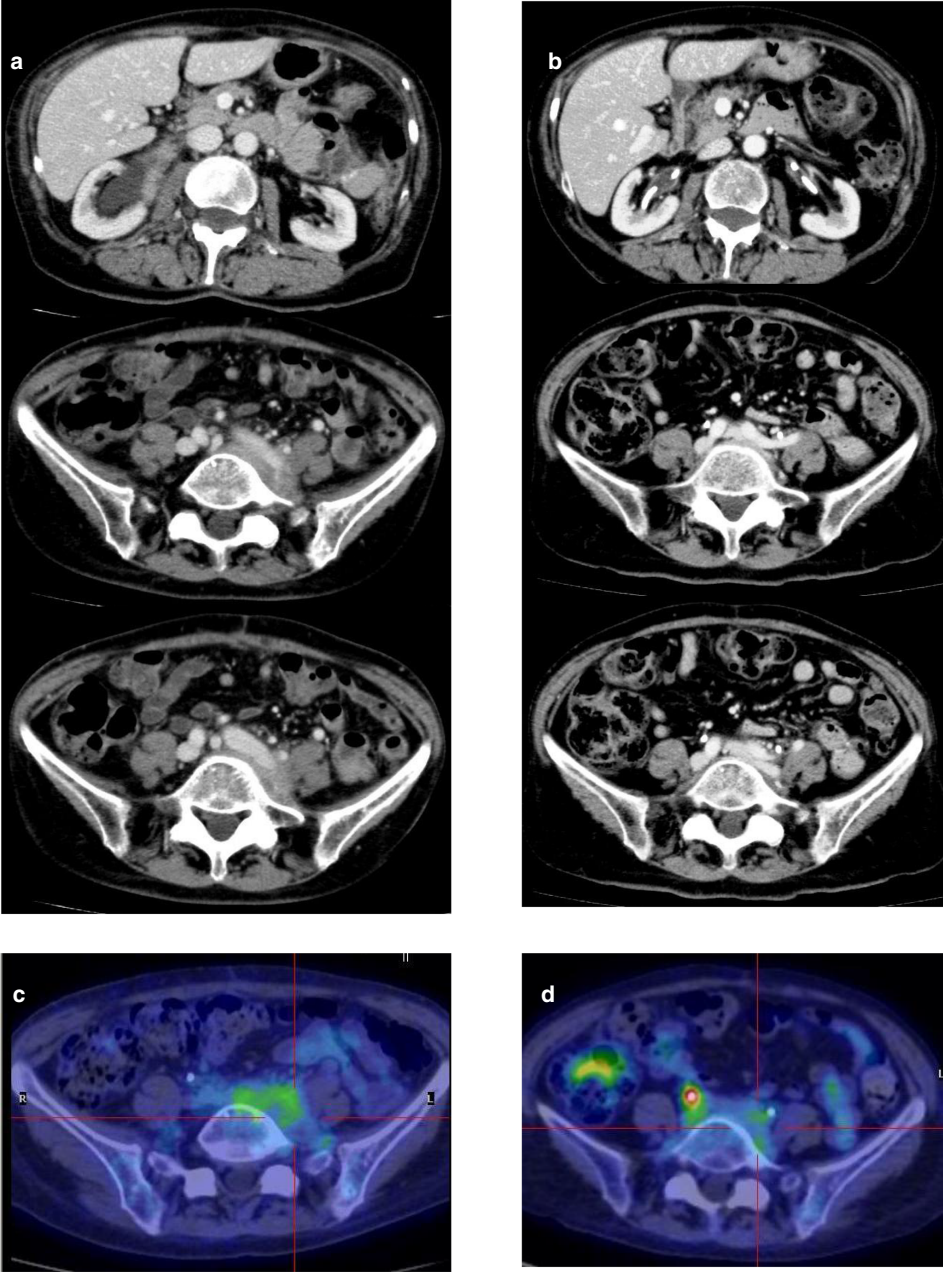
Radiologic features of patient #5. CT scan and PET/CT imaging before starting therapy (a, c) and at 6 months (b, d): a retroperitoneal and perivascular fibro-inflammatory tissue, with (c) high metabolic activity; b complete disappearance of abnormal tissue, with (d) persistence of residual minimal pelvic metabolic activity
